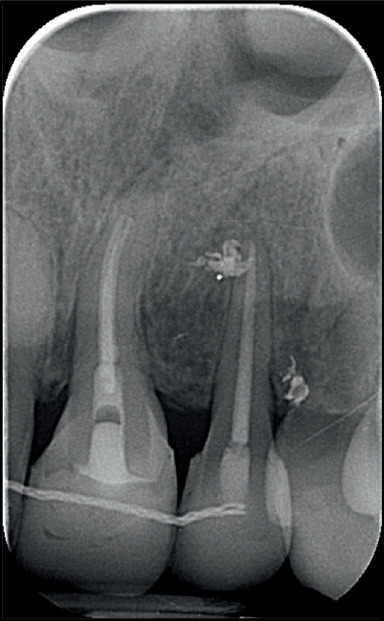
Radiographic follow-up after 8 and 7 years since the autotransplantation of Teeth 2.1 and 2.2, respectively.
